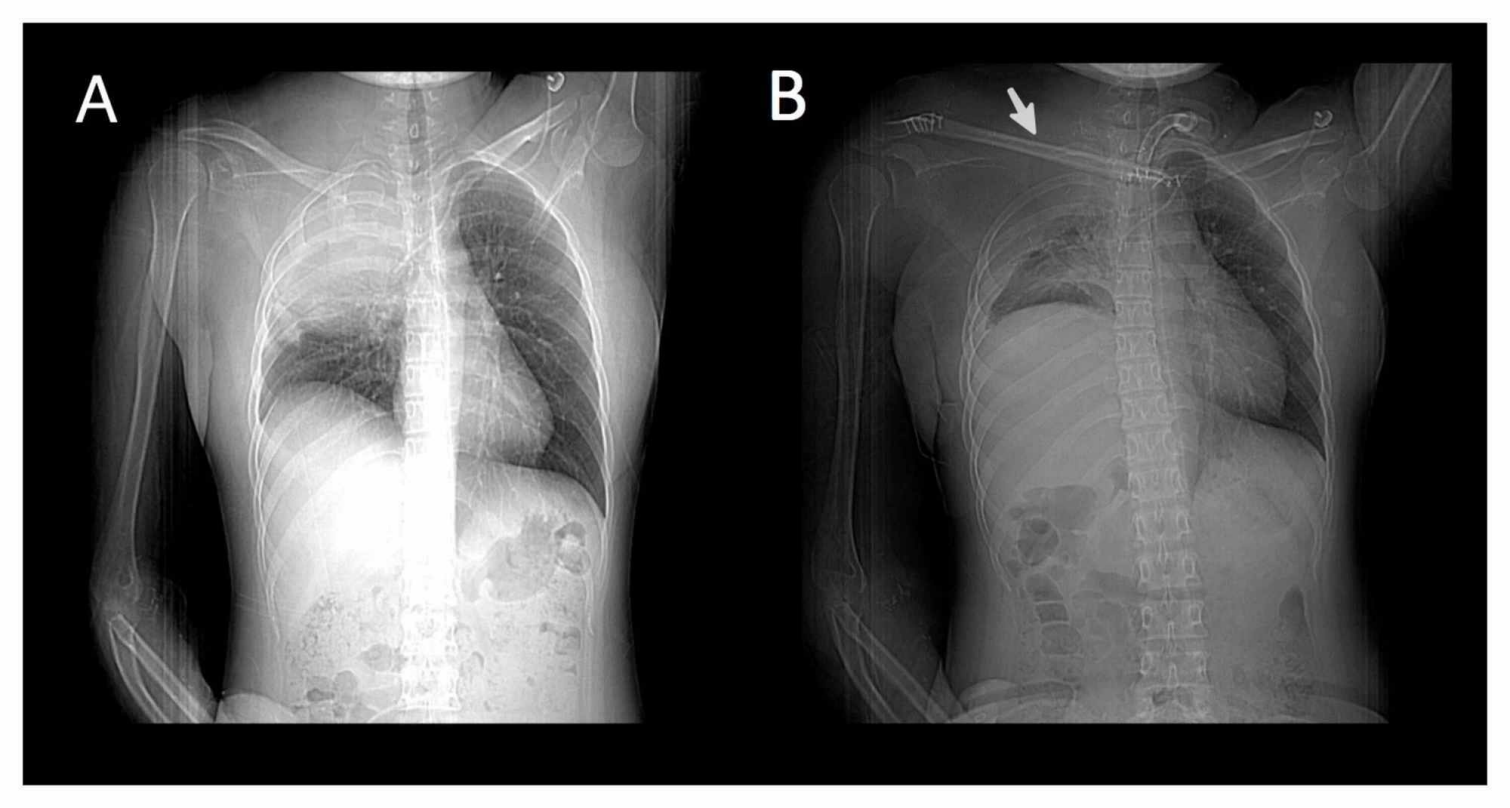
Chest X-ray. (A) Preoperative and (B) postoperative, arrow shows fibula osteocutaneous flap.
